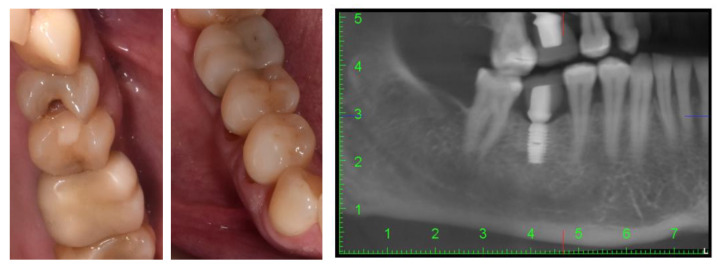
Intraoral aspects and CBCT image of patient.
